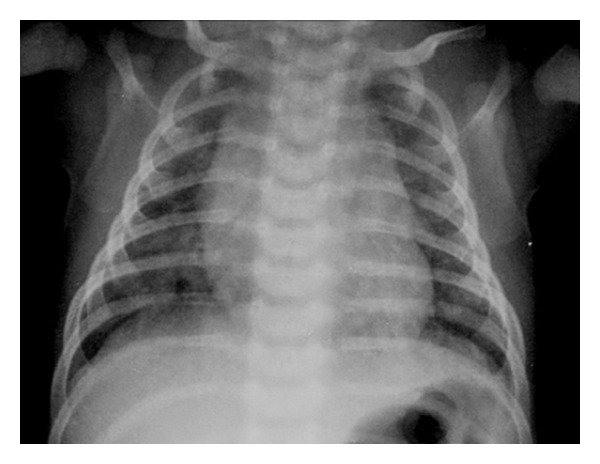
Hyperinflation pattern-chest radiograph showing pulmonary bilateral hyperinflation.
